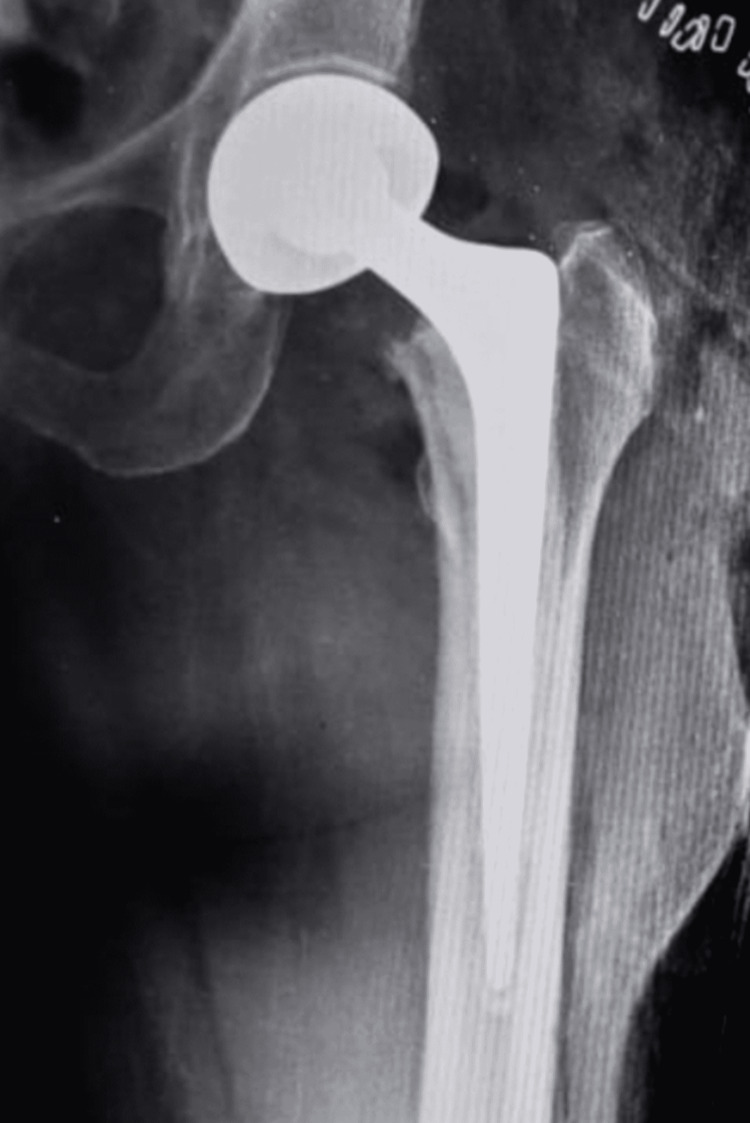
Post-reduction X-ray of the hip X-ray showing reduced head with no other intraoperative events like fractures or loosening.
